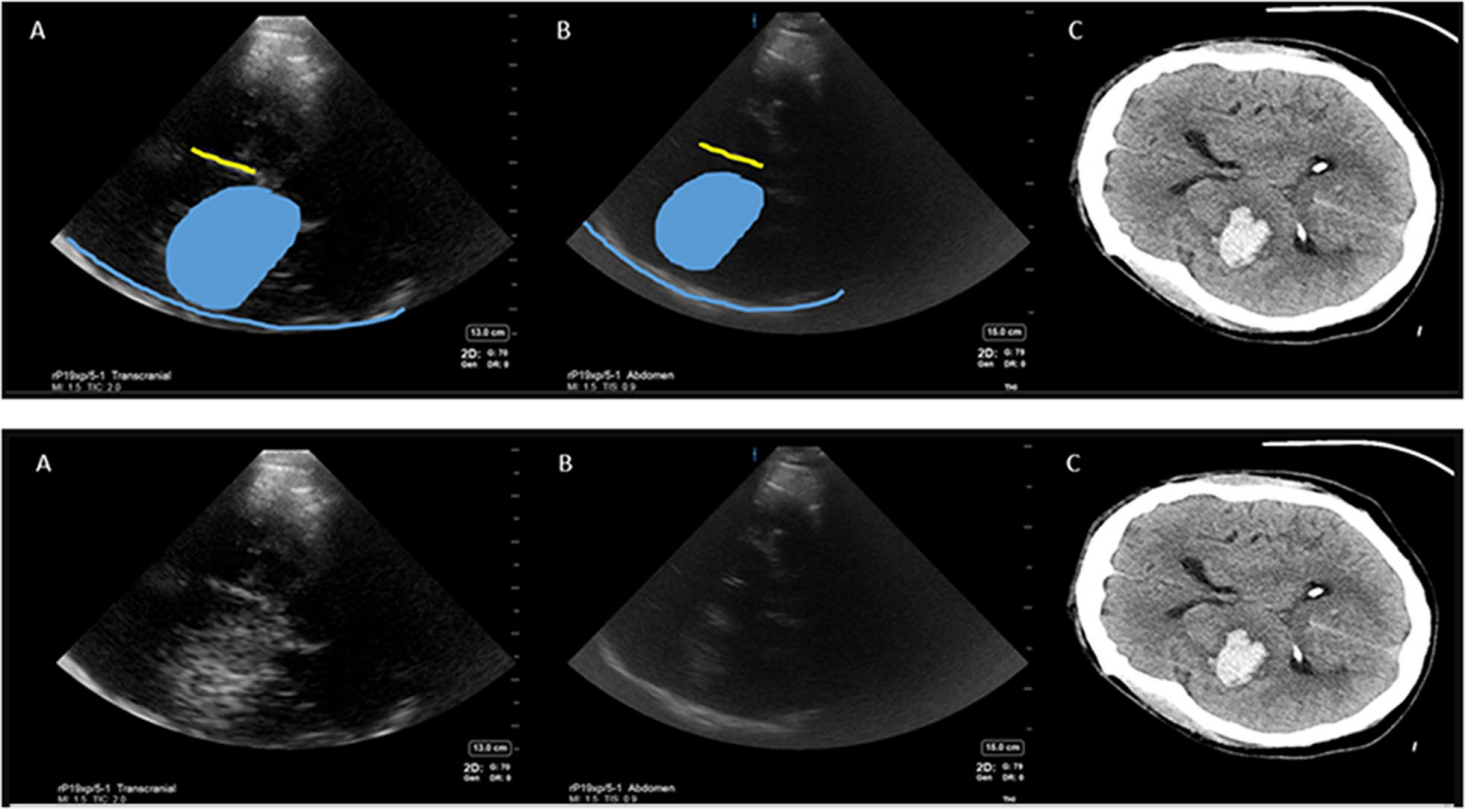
Intracerebral hemorrhage visible as a hyperechoic signal best visualized contralateral to the insonated window. A—Transcranial preset, B—abdominal preset, C—COMPUTED tomography brain (CT) scan. Blue line—opposite skull, yellow line—falx cerebri, blue shape outlines the hyperechoic signal corresponding to hemorrhage on CT scan
